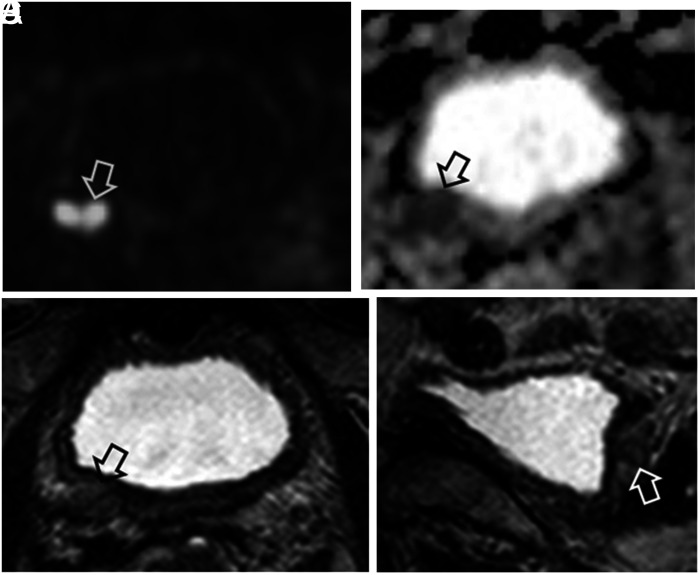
PI-RRADS category 4 based on biparametric MRI, 8 years after RP for prostate adenocarcinoma Gleason 7 score, in 74-year-old patient with PSA increased by 2.86 ng/mL. The lesion detected as hypointense on DWI at high *b* value (arrow in A) and inhomogeneous moderately/markedly hypointense on ADC map (arrow in B) and inhomogeneous on T2W images (arrow in C). The lesion is located at the level of the right posterior wall of the bladder cranially 15 mm above a plane passing through the lower margin of the pubic symphysis (arrow in D).
